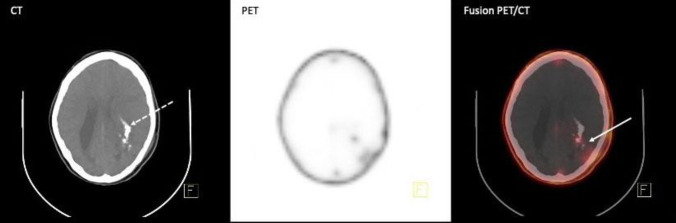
Brain [^68^Ga] Ga-Pentixafor PET/CT. mild CXCR4 avidity (SUV_max_: 1.68) in the left occipito-parietal lobe (solid arrow) with evidence of adjacent calcification (dotted arrow)
